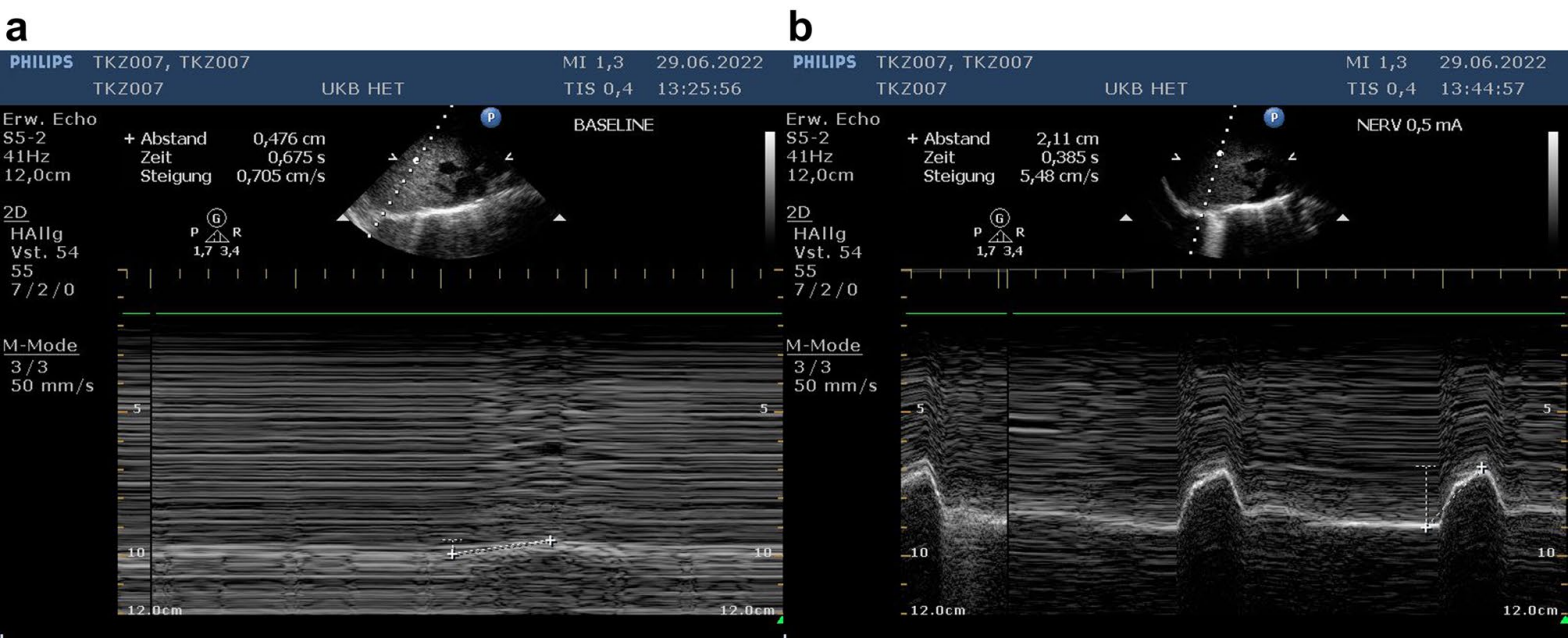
Ultrasound examination of the externally stimulated right phrenic nerve. (a) Minimal diaphragmatic excursion without stimulation during the inspiratory cycle of the respirator. (b) Regular diaphragmatic excursion following external stimulation of the nerve with a stimulus of 0.5 mA.
